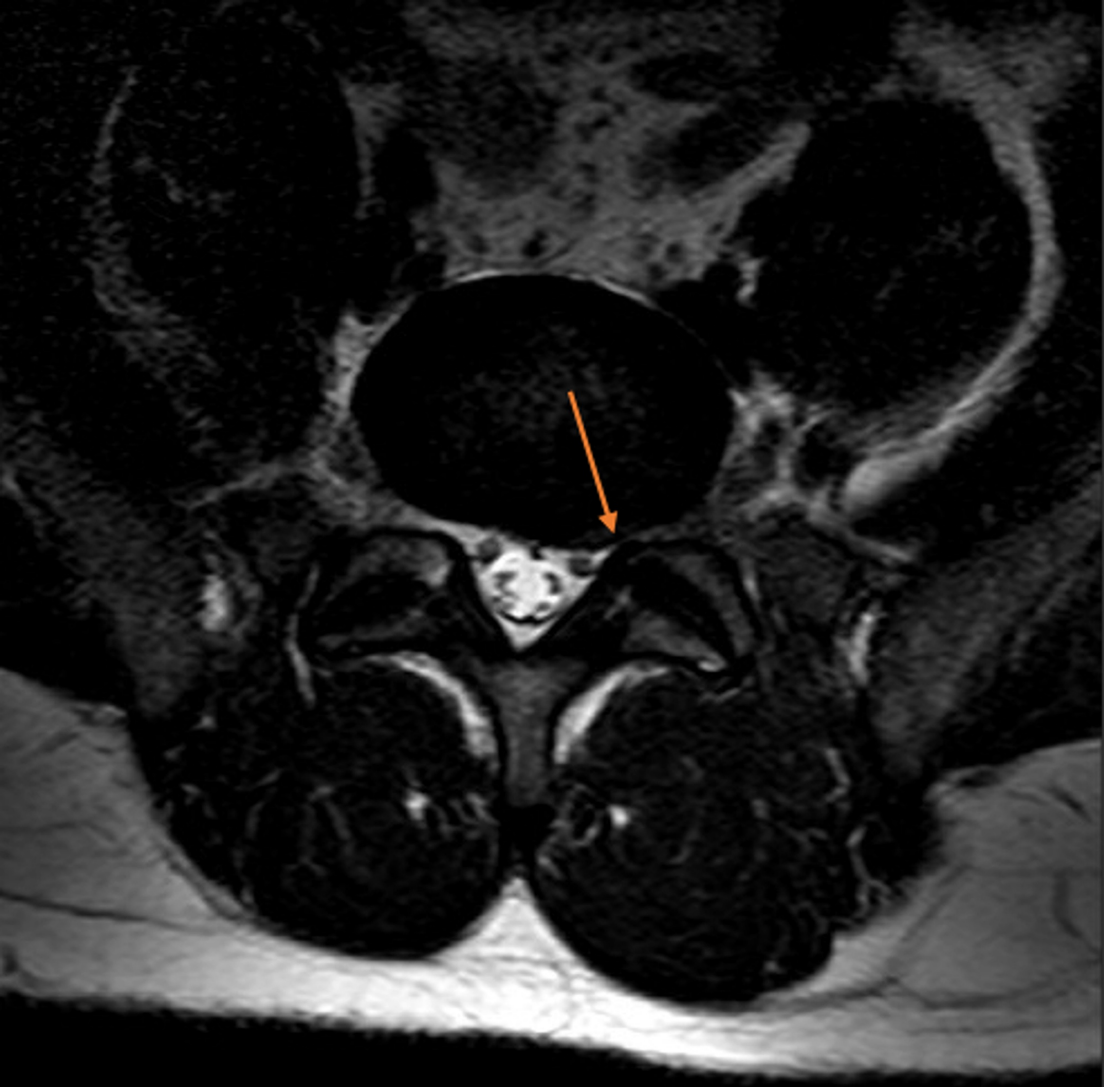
MRI of the lumbar spine showed degenerative retrolisthesis of L5 on S1 with a degenerative L5-S1 disk cartilage and with a small left-sided disk protrusion (axial view).
